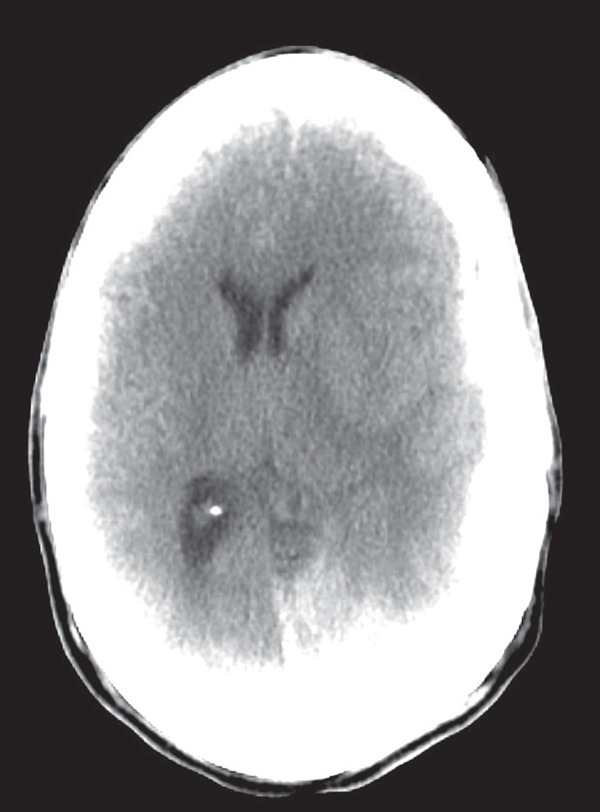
CT brain without contrast, coronal view.
